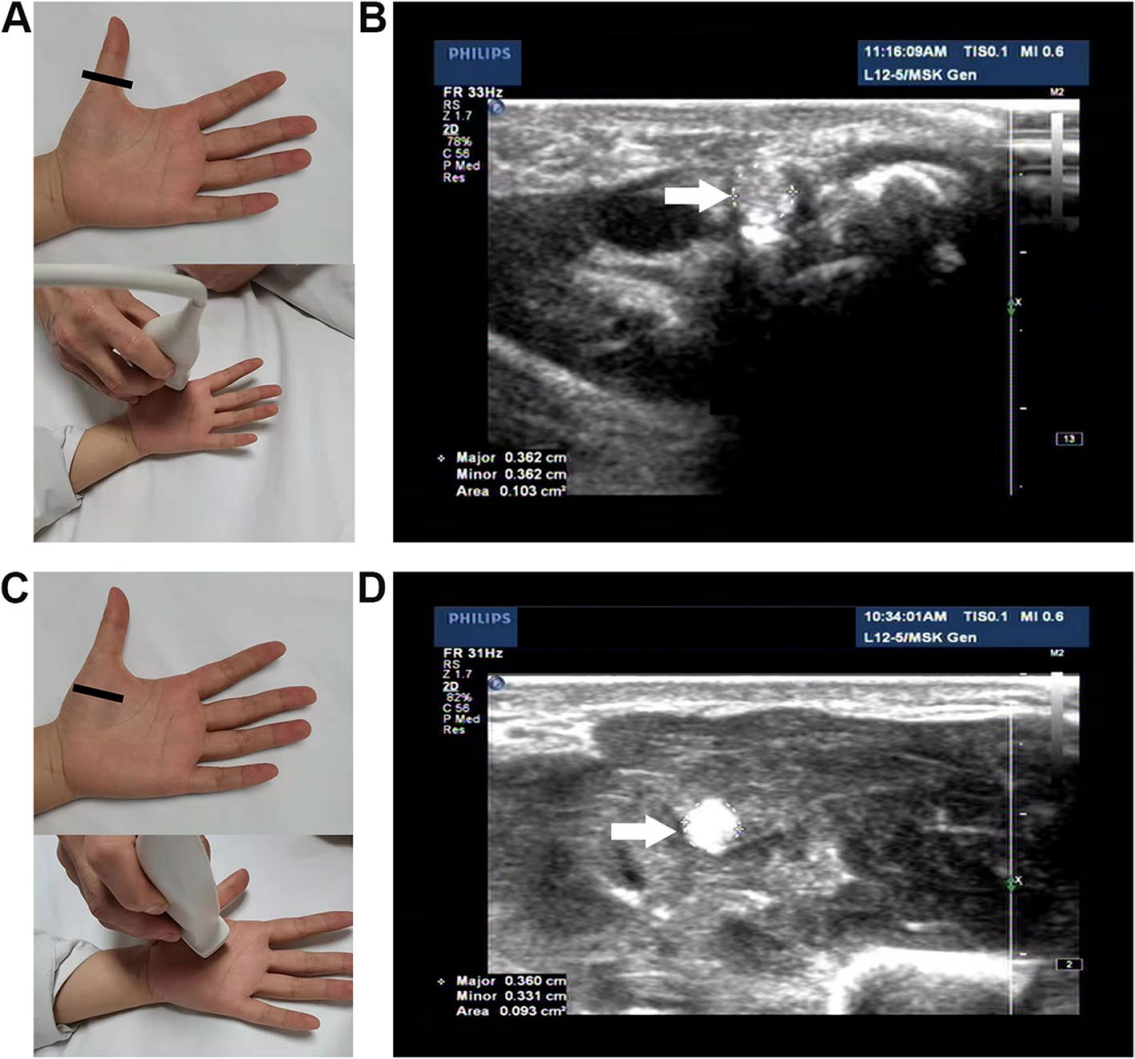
A-B Ultrasonic probe position (black line) and ultrasonic measurement image (dotted line) of the cross-sectional area (C1) of FPL tendon (white arrow) at the MCP joint. C-D Ultrasonic probe position (black line) and ultrasonic measurement image (dotted line) of the cross-sectional area (C2) of FPL tendon (white arrow) at the midpoint of the first metacarpal bone
